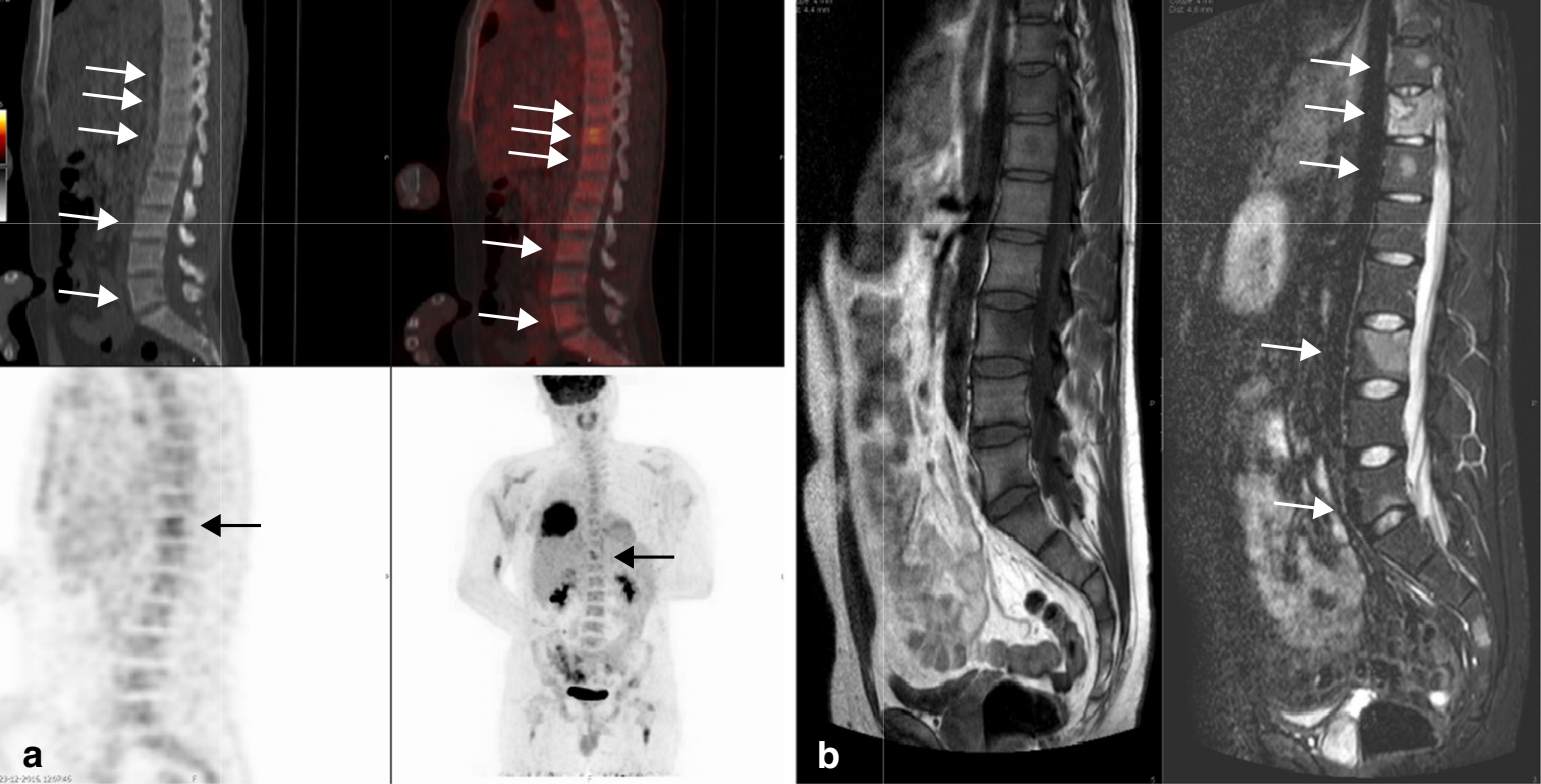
False-negative lesions on ^18^F-FDG PET-CT (*arrows*). A 23-year-old man presenting with metastatic Ewing sarcoma of the right seventh rib. Images obtained at diagnosis, before the start of treatment. a^18^F-FDG PET-CT showing increased ^18^F-FDG-uptake at the eleventh thoracic vertebrae only. b T1-weighted (*left*) and STIR (*right*) images showing nodules with a high degree of suspicion for metastasis at the tenth, eleventh, and twelfth thoracic vertebrae and the third and fifth lumbar vertebrae
